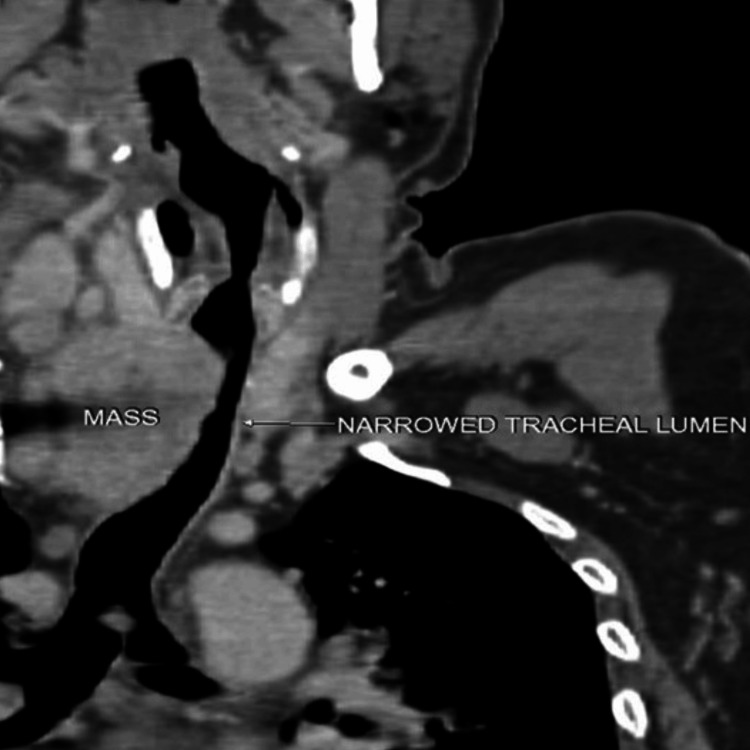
Sagittal section of PET-CT showing narrowed tracheal lumen at C7-D1 vertebral level.
